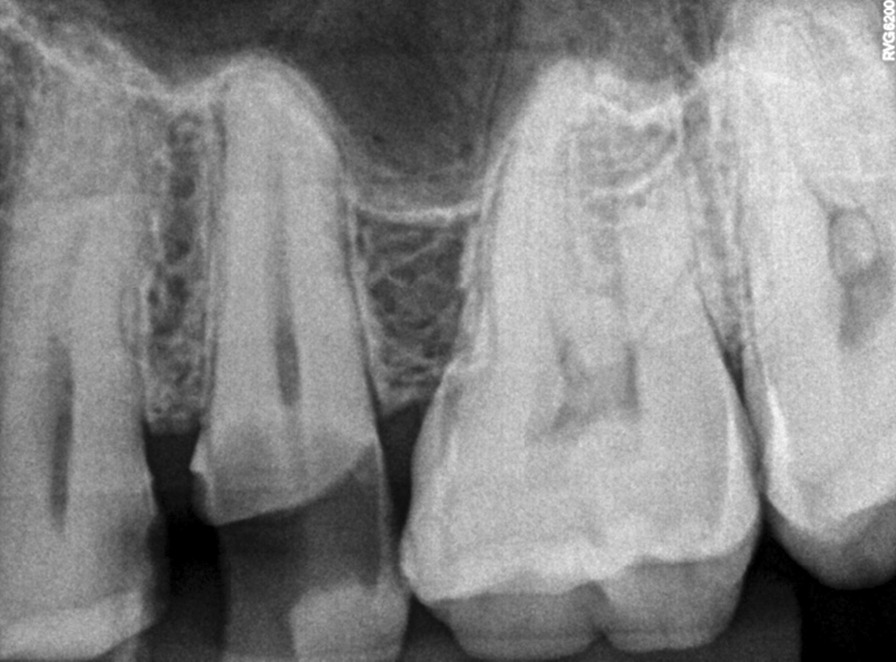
Radiograph of the tooth represented in Fig. 2A as sample ‘A’
